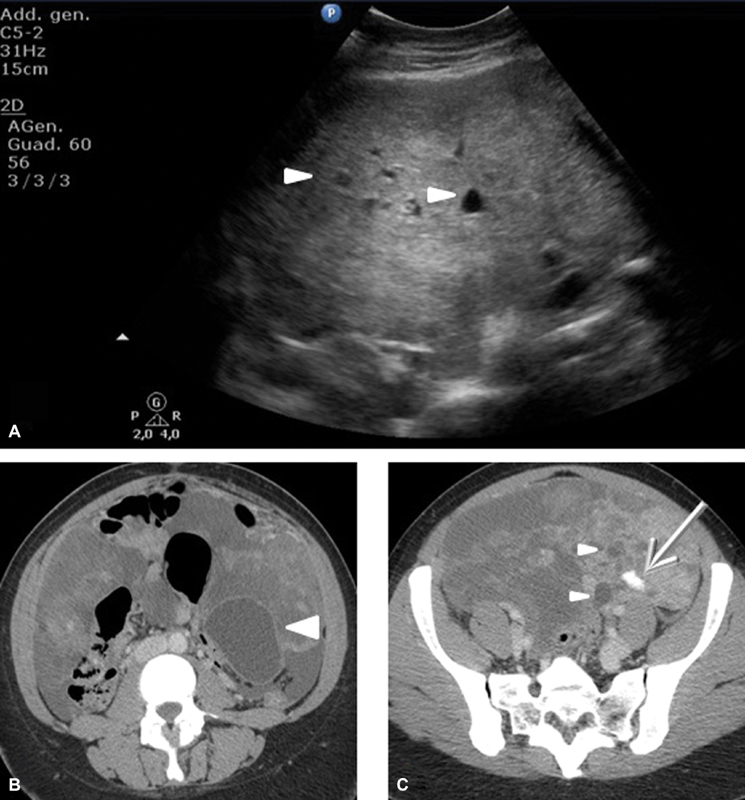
Transabdominal ultrasound (A) and axial CECT (B-C) images show a large solid, heterogeneous mass containing cystic spaces (▸). Note the presence of calcifications (→) (C).
